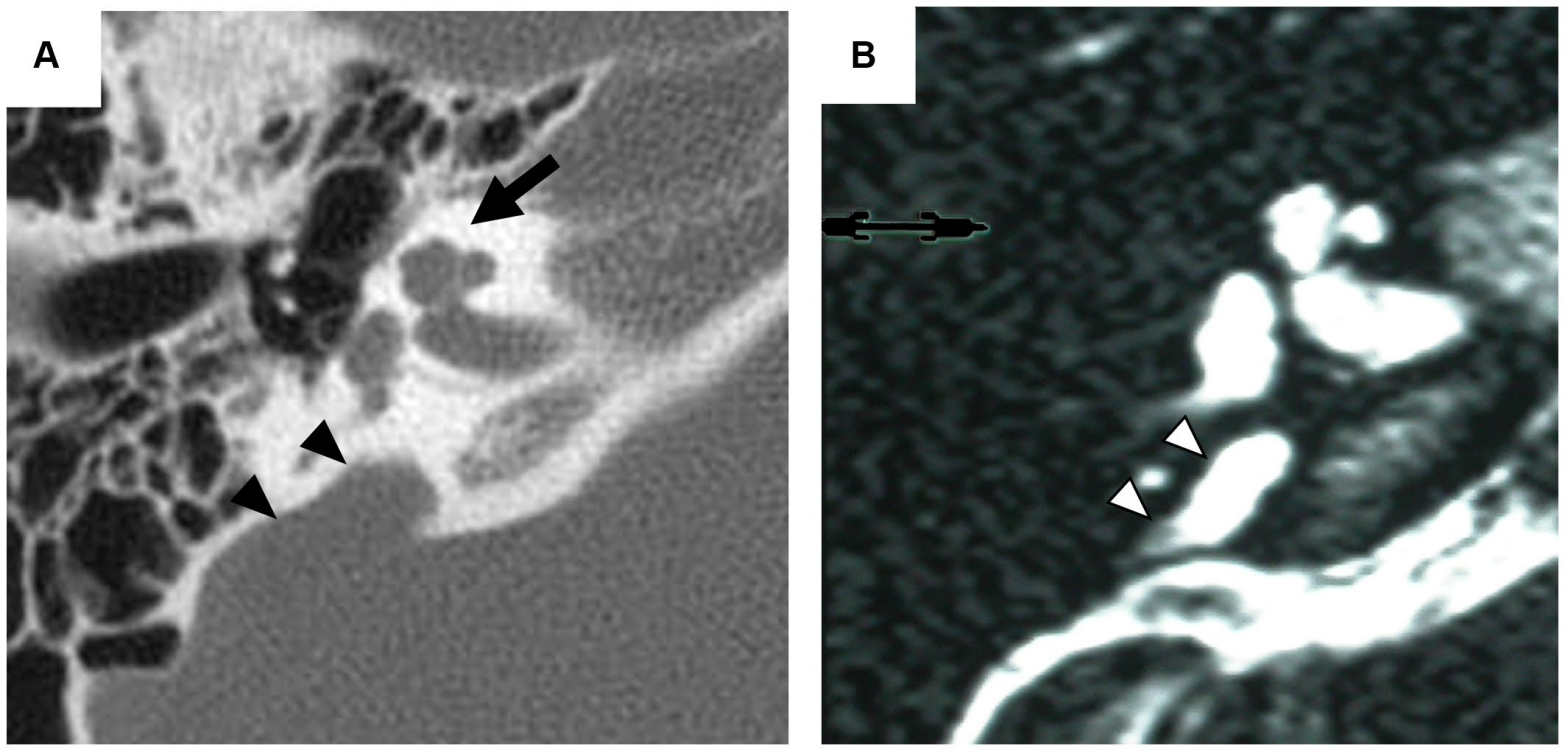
Radiographic imaging of the inner ear in a patient with incomplete partition type II. Axial computed tomography (CT) scan (A) exhibits the cystic appearance of the apical turn of the cochlea (arrow) and the enlarged vestibular aqueduct (arrowhead). Axial T2-weighted magnetic resonance (MR) image (B) provides an enlarged endolymphatic duct (arrowhead).
